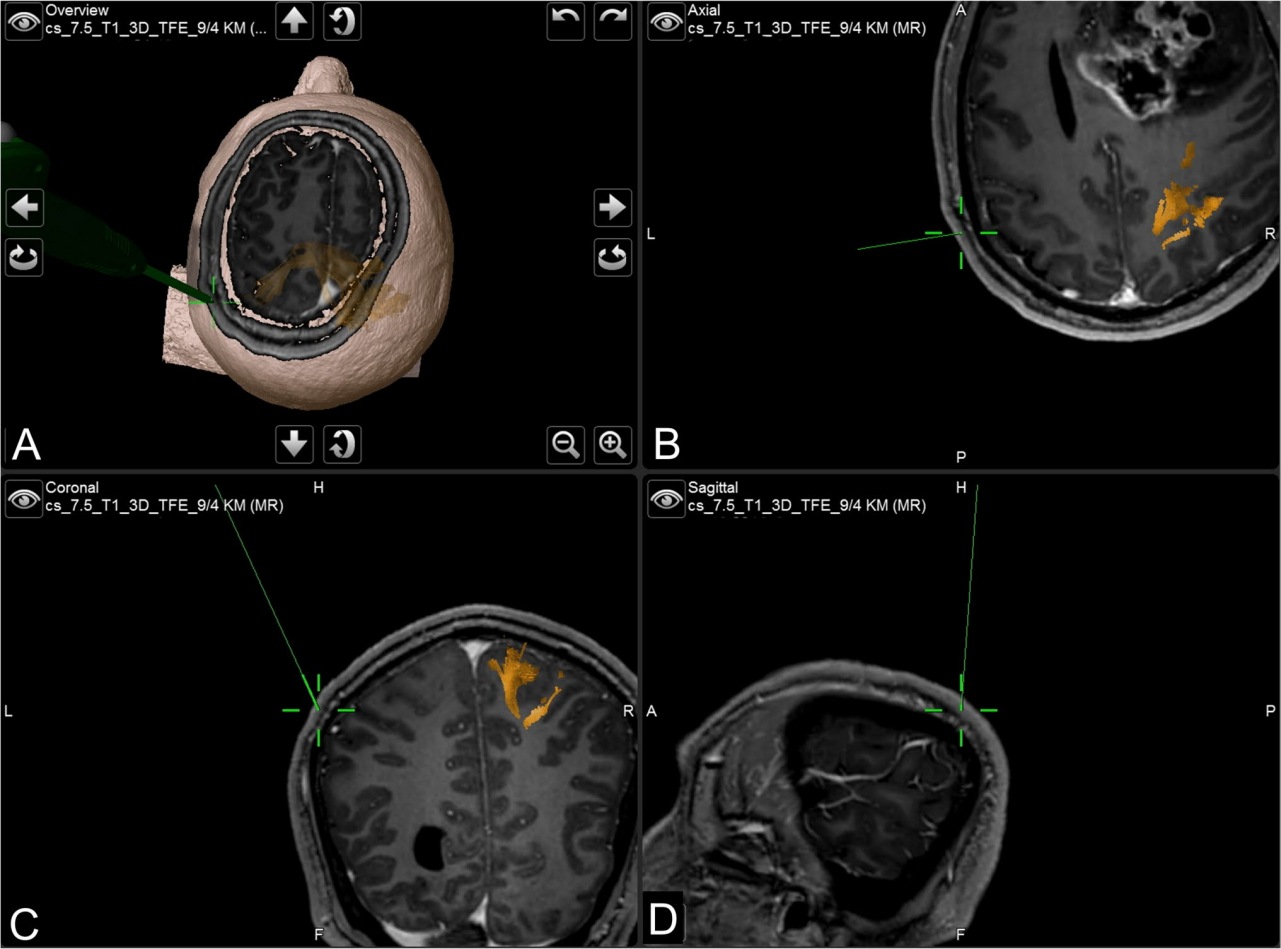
Screenshot of the navigation system. Navigational guide instrument pointing to the left parietal lobe for placement of a transcranial stimulation electrode (A). In axial (B), coronal (C), and sagittal (D) reconstructions of a T1-weighted MRI. Corticospinal tract fibers of contralateral side highlighted in yellow according to preoperative functional nTMS mapping
